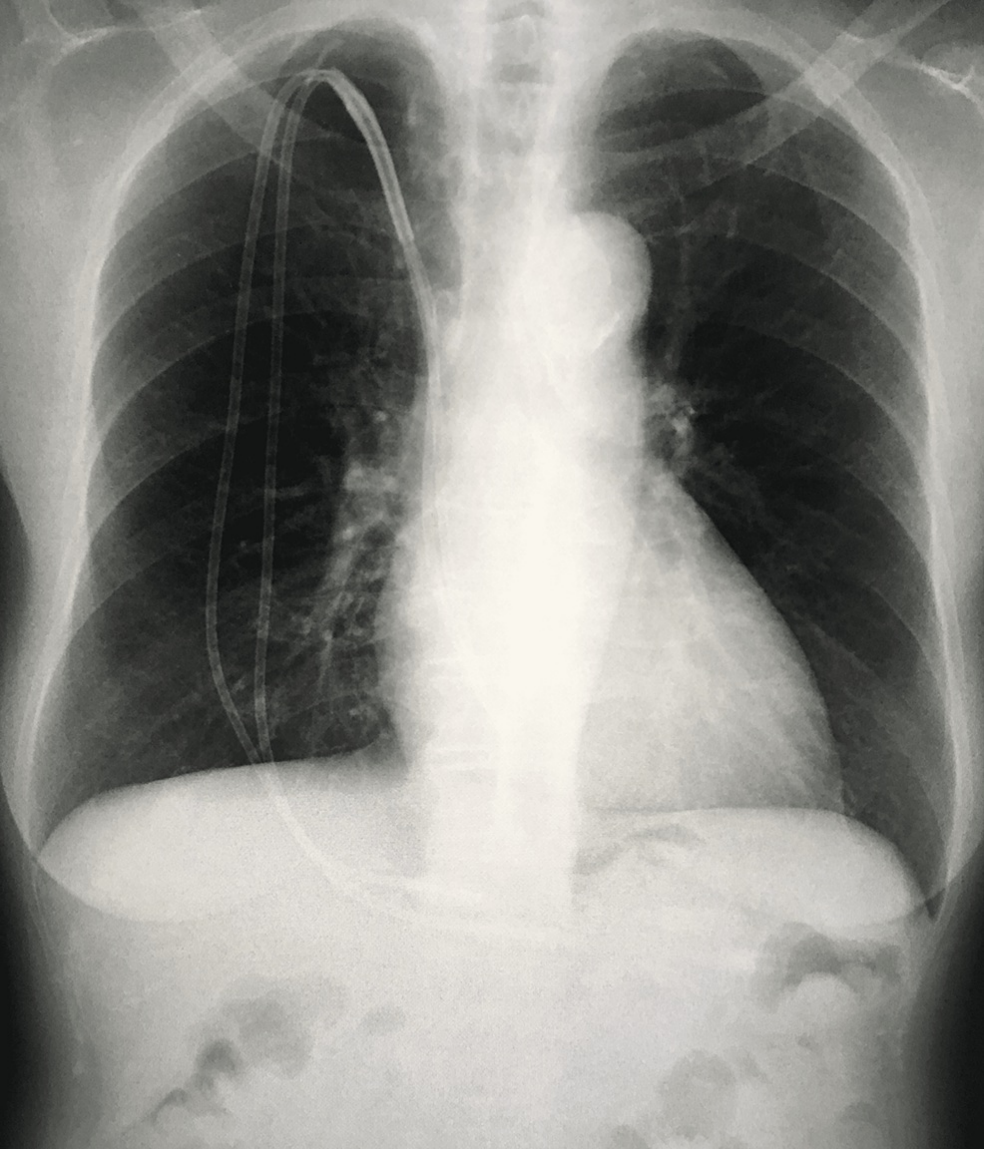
Chest radiograph showing the tunneled cuffed hemodialysis catheter inserted into the right subclavian vein.
